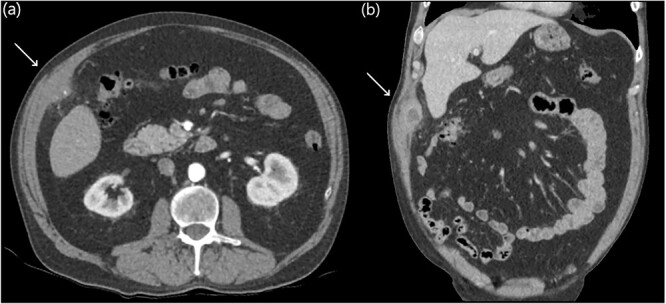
Abdominal CT axial slices (a) demonstrating a tiny focal calcific density in the right upper quadrant, and coronal slices (b) demonstrating a right upper quadrant intramuscular collection.
